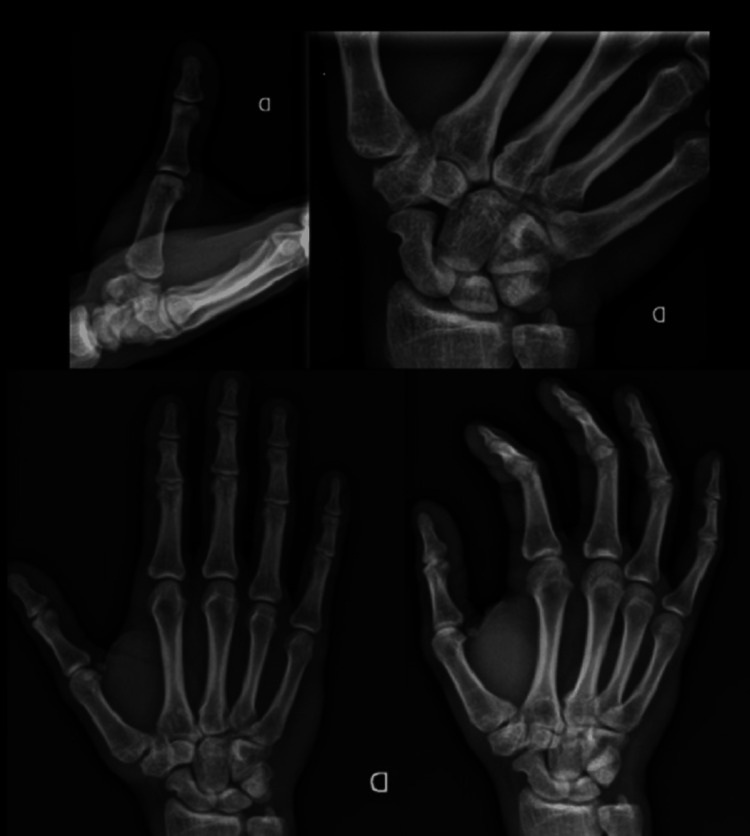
Radiographs with different views of the right hand at the five-year follow-up showing minimal degenerative changes of the first trapezio-metacarpal joint.
